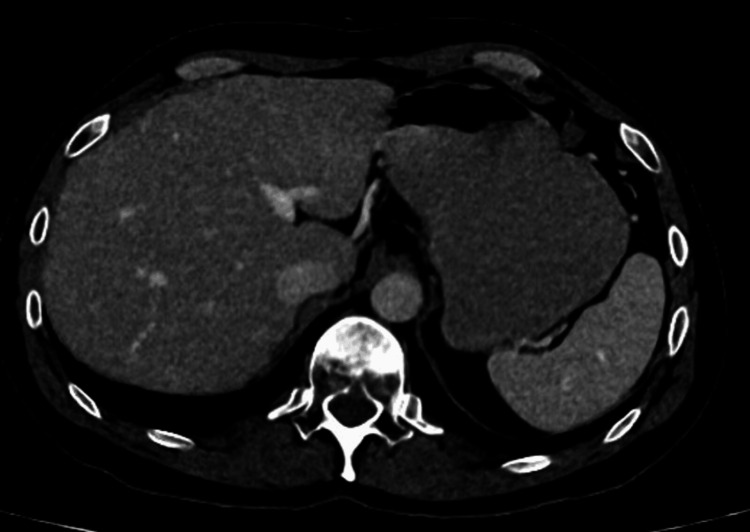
CT scan showing liver metastases after 12 months on alectinib CT: computed tomography
